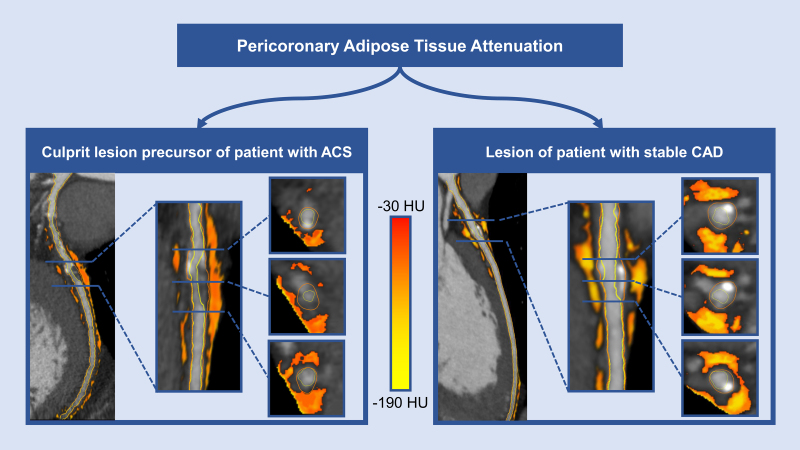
Pericoronary adipose tissue (PCAT) attenuation on computed tomography angiography (CCTA). Multiplanar reconstructions of CCTA scans showing mixed plaques in the proximal (right) and middle (left) left anterior descending coronary artery, with surrounding pericoronary adipose tissue (orange-yellow colored areas) across a precursor of a culprit lesion of a patient who developed an ACS (left) and across a lesion of a patient with stable CAD (right). ACS indicates acute coronary syndrome; CAD, coronary artery disease; and HU, Hounsfield units.
